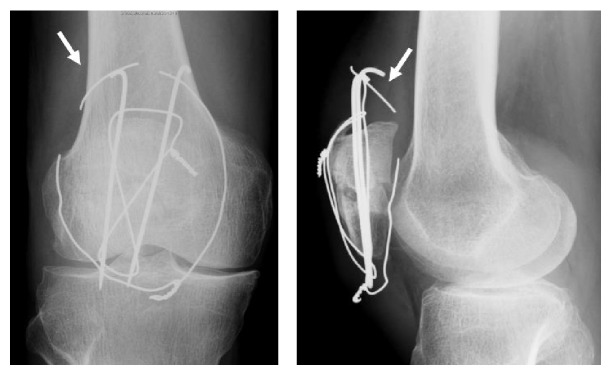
Plain radiographs at 10 weeks postoperatively show breakage of the superior portion of the cerclage wire (arrow).
